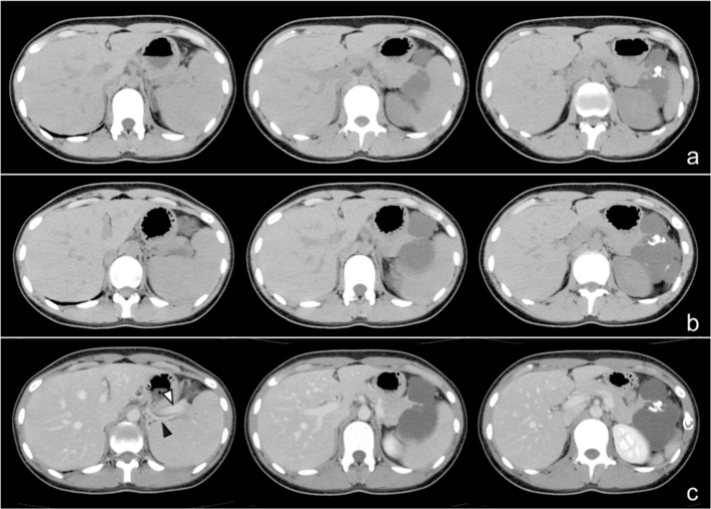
Enhanced CT scan showed that the tumor size increased to 5.1 cm in diameter for 6 years (a) and in the subsequent 3 months increased to 6.3 cm eventually (b). Its septum and capsule are not enhanced, and both splenic artery (*black arrow head*) and splenic vein (*white arrow head*) are not invaded by the tumor (c)
